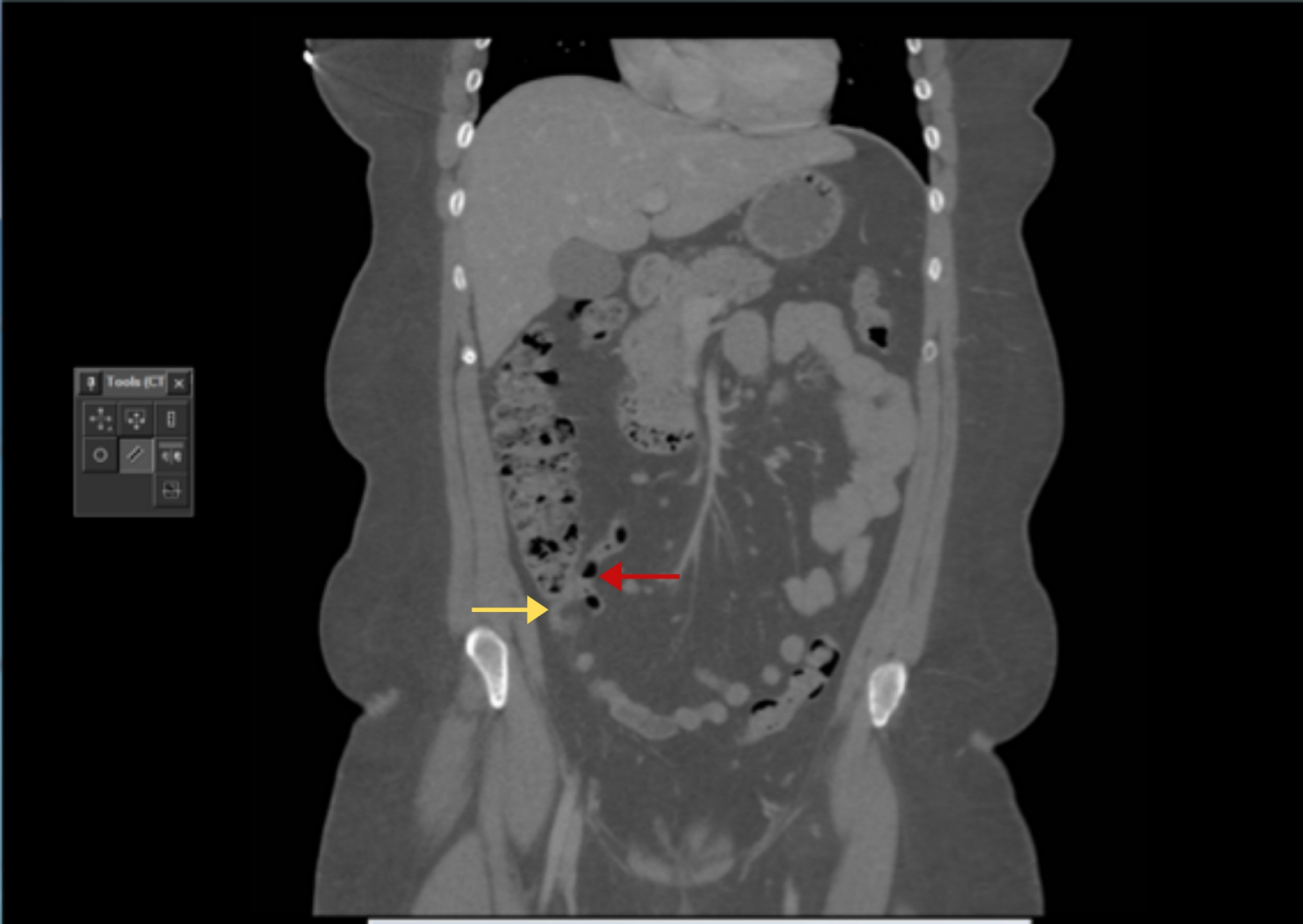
Coronal section of the CT scan showing a normal appendix (red arrow) with some fat stranding around the appendix (yellow arrow)
